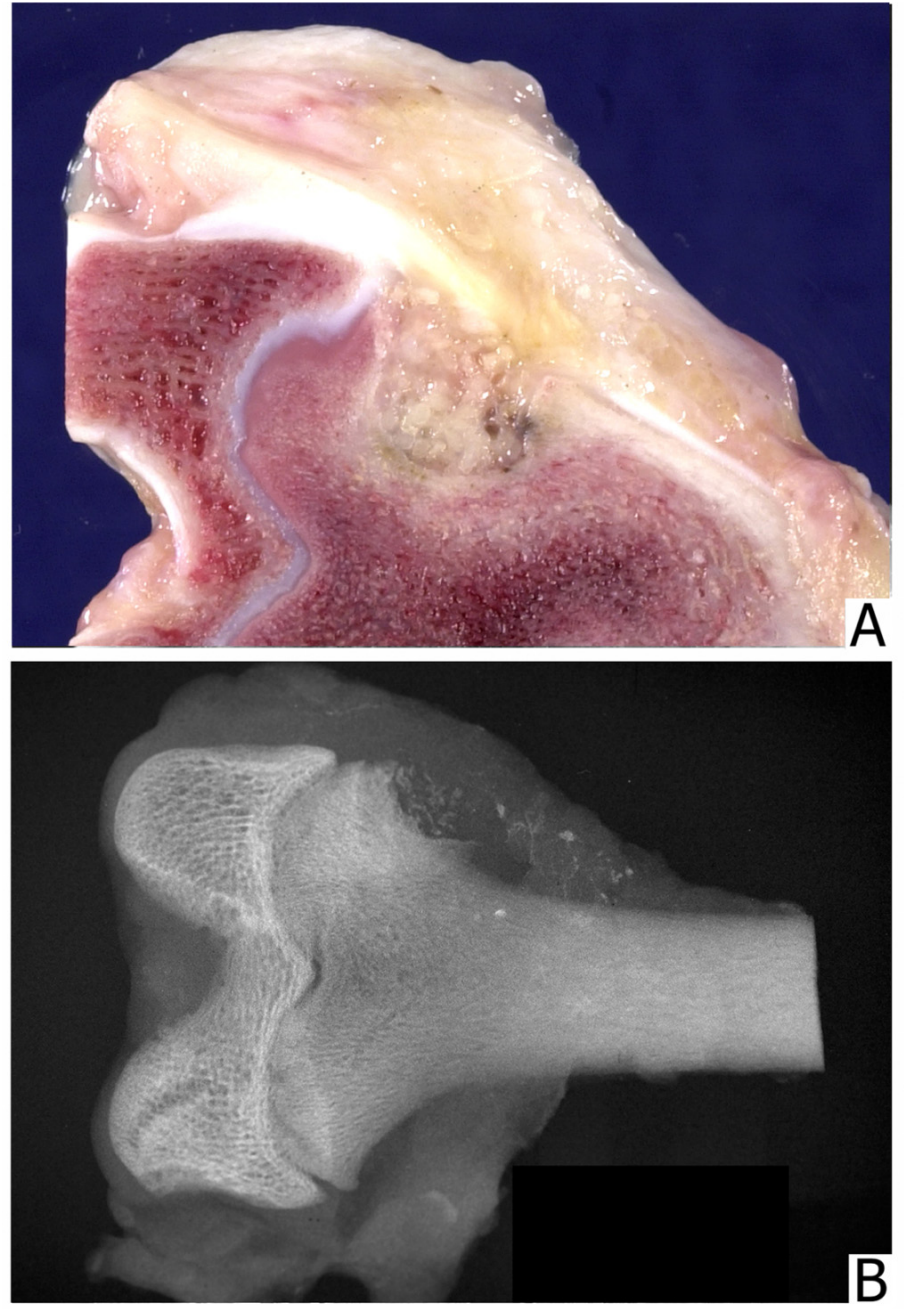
(A) Defect filled with 1-2 mm bone particles; particles are recognizable grossly. (B) A radiograph of a defect filled with 800-500 µm particles. The edges of the defect are distinct and the granules are recognizable.
